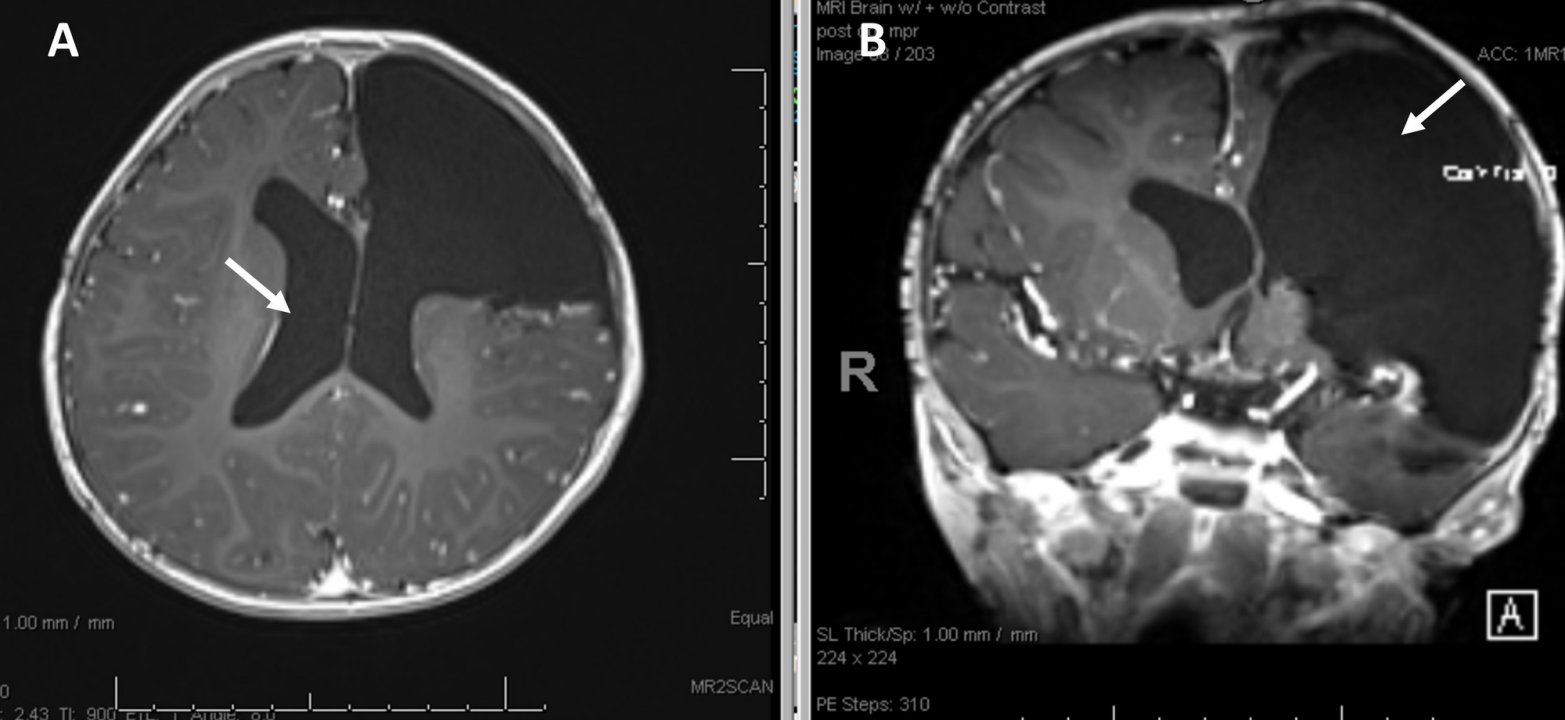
Imaging post second resection and continued chemotherapy MRIs status-post 15 months from the initial resection and 12 months post the second resection. (A) Axial MRI showing resolution of hydrocephalus (arrow). (B) Coronal MRI showing post-operative changes with no residual tumor (arrow)
